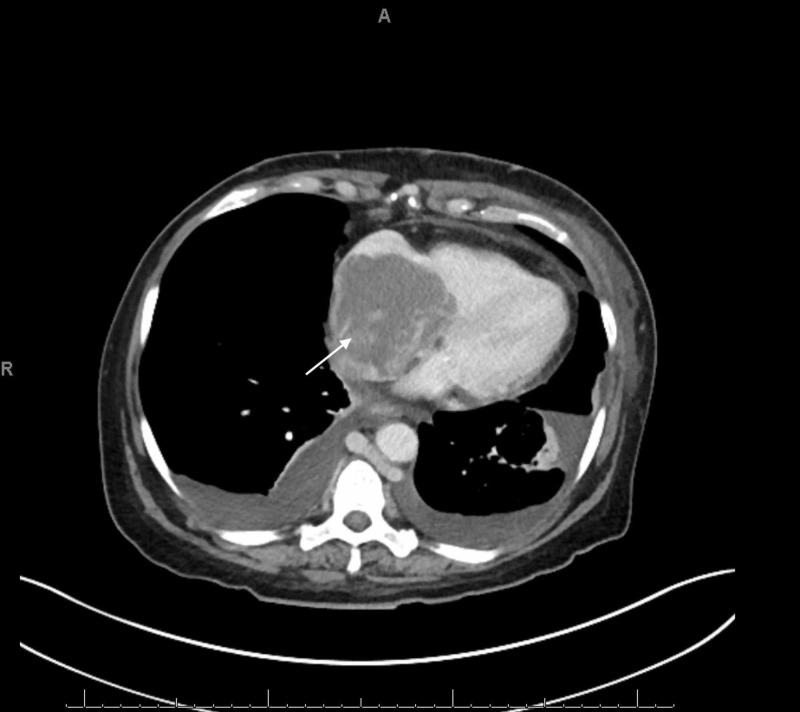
Triple-Phase Liver CT - Transverse Section of the Right Atrium Transverse view of triple-phase liver CT revealed a mass in the RA with tricuspid involvement and its own vascular supply.
